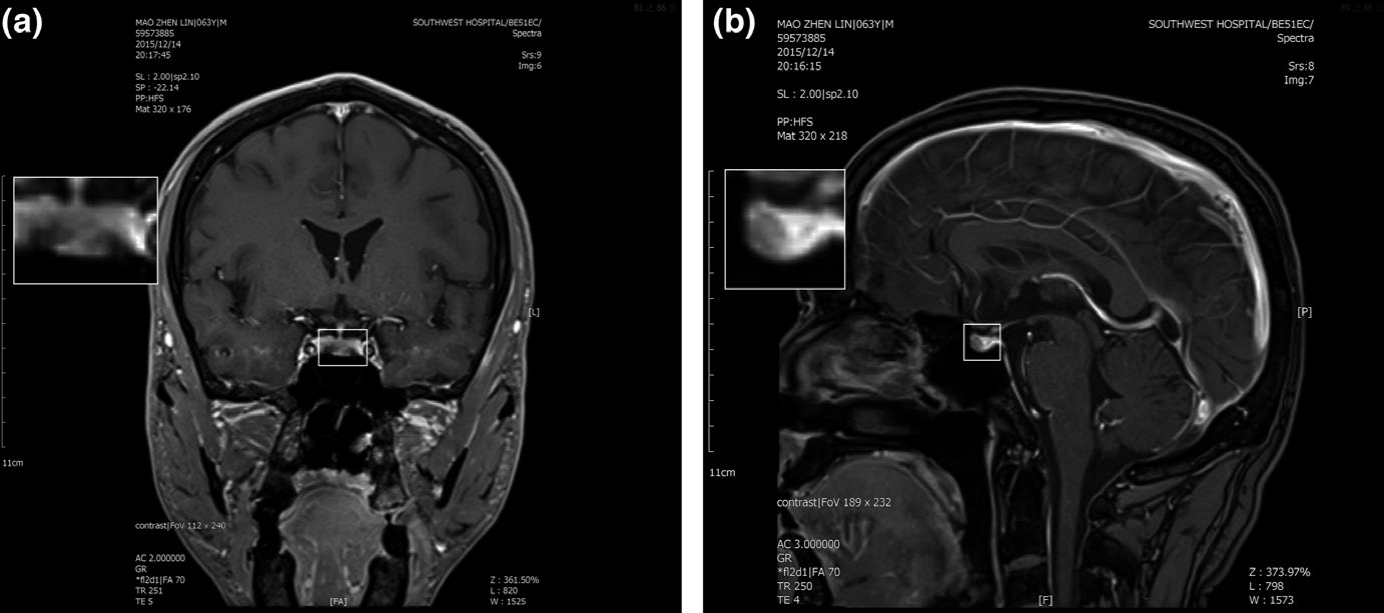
Pituitary enhanced MRI scan of case one showed a small round low signal shadow
